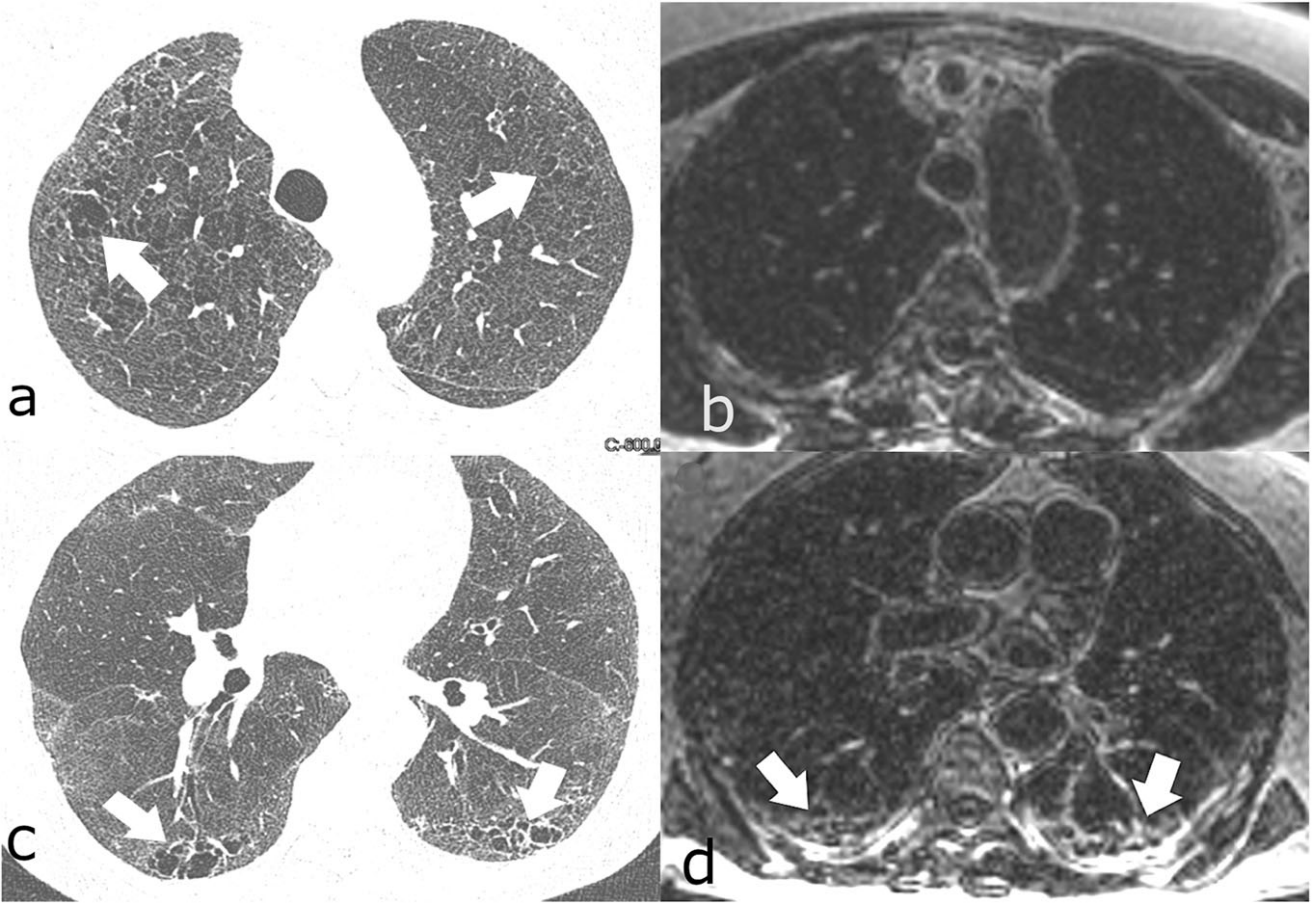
Seventy-two-year-old woman with progressive breathlessness, FEV_1_ 2.4 L/s, LAV 1.5%. a Axial high-resolution computed tomography showed mild centrilobular emphysema in the upper lobes (arrows). b It was unclear at multidisciplinary conference whether the abnormal regions in the lower lobes (arrows) represented emphysema or fibrosis. c Late gadolinium-enhanced magnetic resonance imaging of the upper lobes showed no contrast enhancement, but in d, definite contrast enhancement was observed in the abnormal regions in the lower lobes (arrows) and was consistent with fibrosis. *FEV*_*1*_, Forced expiratory volume in one second; *LAV%*, Low attenuation value percent
